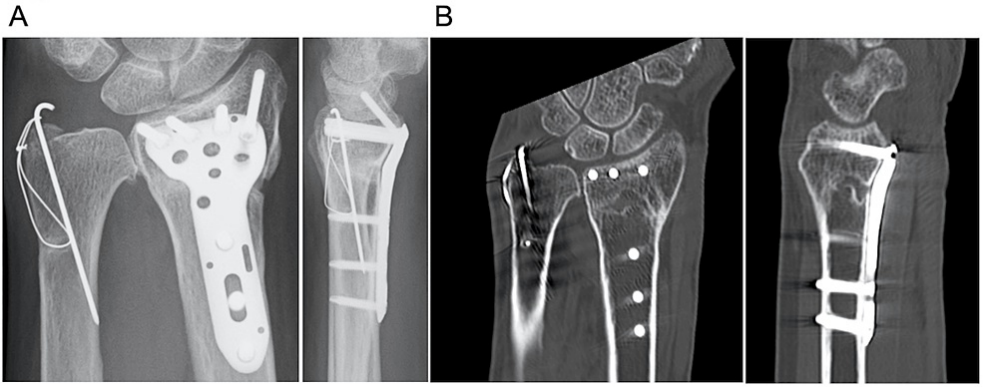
(A) Posteroanterior and lateral radiographs of the left wrist 5 months after the application of low-intensity pulsed ultrasound (LIPUS). (B) Coronal and sagittal computed tomography images 5 months after the application of LIPUS.
